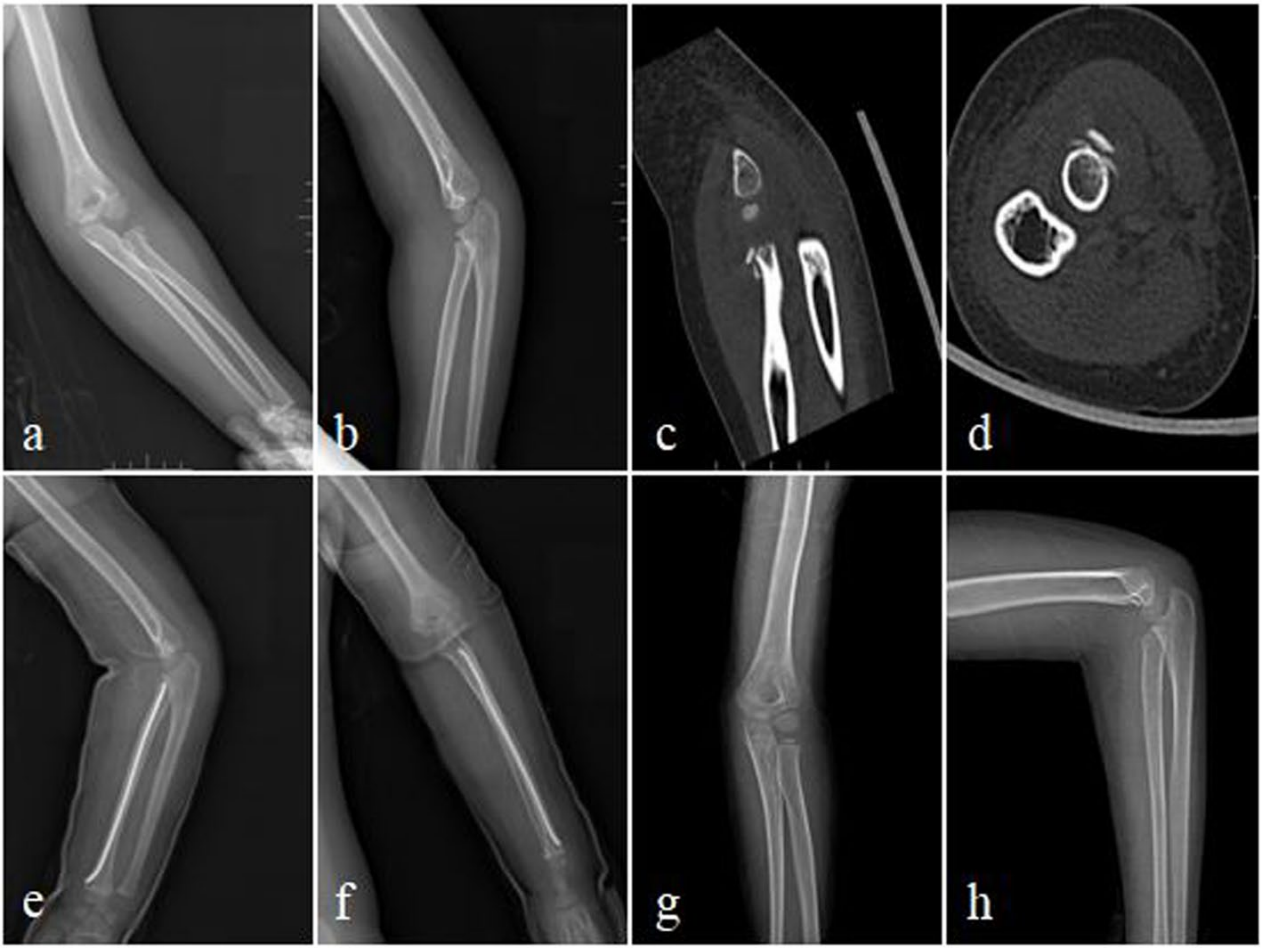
A four-year-old girl presented with left radial neck fracture. a-d Preoperative X-ray and 3D CT examination. e, f X-ray on the first day after surgery. g, h X-ray at the final follow-up
